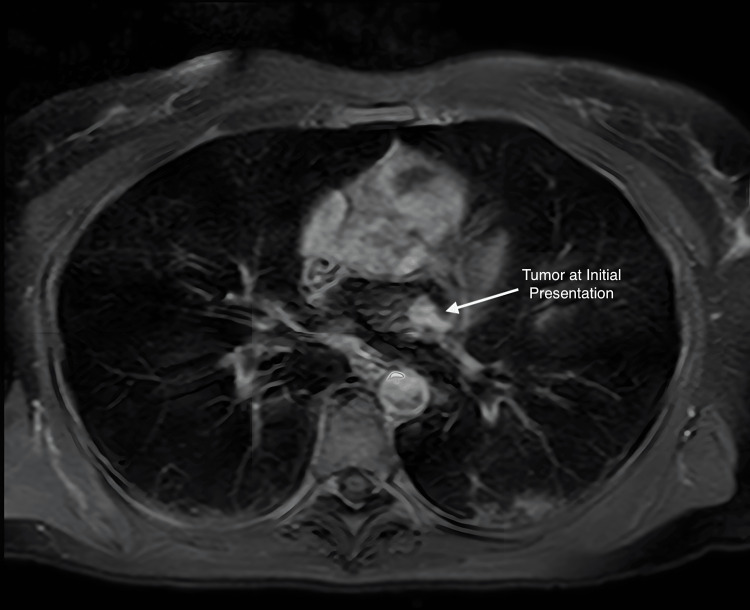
Axial MRI scan of the heart demonstrating a 1.3 cm × 1.2 cm mass in the left atrium along the supero-lateral wall.
